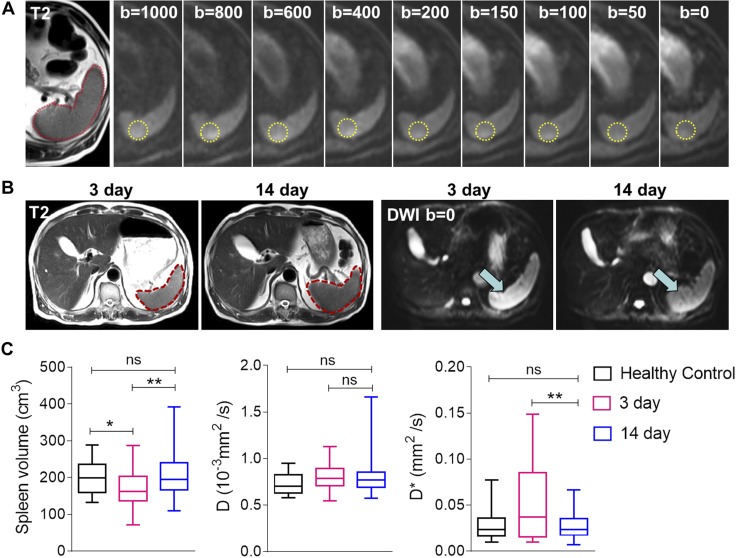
Spleen shrinkage and increased capillary perfusion after ICH onset. *A*) Representative images of abdominal MRI with T2-weighted images and DWI at 9 consecutive *b* values from 0 to 1000. Spleen volumes were calculated from T2 images. Red outlines show the spleen, and yellow circles show the ROI for measuring signal intensity of the spleen from DWI images at different *b* values—signal density decayed with increasing *b* values. IVIM-DWI parameters, *D* and *D, were derived from these multiple DWIs. *B*) Representative abdominal MRIs from a patient with ICH at d 3 and 14 after ICH show an enlarged spleen at d 14 compared with d 3 (spleen is outlined in red; right). DWI images at *b* = 0 of the same patient show that the signal density of the spleen at d 3 was higher than at d 14, which indicates increased perfusion at d 3 post-ICH (left). *C*) Quantitative analysis shows dynamic changes of spleen volume after ICH onset. *D* and *D values of spleen are compared; the horizontal line inside each box indicates the median. The top and bottom of the box indicate the interquartile range, and error bars indicate the 5th and 95th percentiles. Comparisons were performed by using the Mann-Whitney test. Ns, not significant. P* < 0.05, *P* < 0.01.
